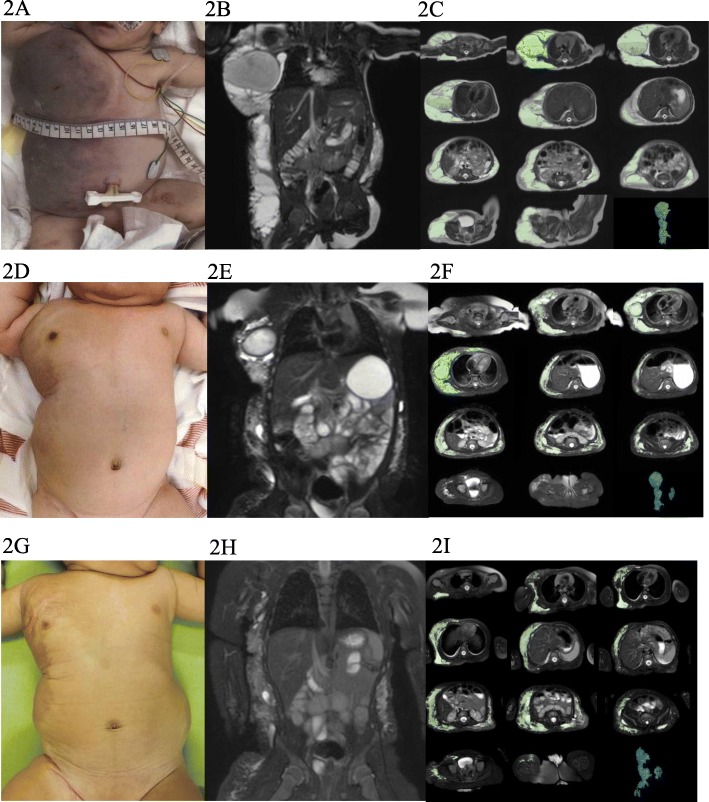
Clinical photograph, MRI, and volumetric measurements for case number 2. A 2-week-old girl had a giant cystic LM lesion in her right neck, axilla, trunk, and abdominal cavity. a-c Pretreatment. d-f 3 months after administration of sirolimus. g-i 6 months after administration of sirolimus. c, f, and i show volumetric measurements evaluated using the Digital Imaging and Communications in Medicine (DICOM) viewer (OsiriX)
